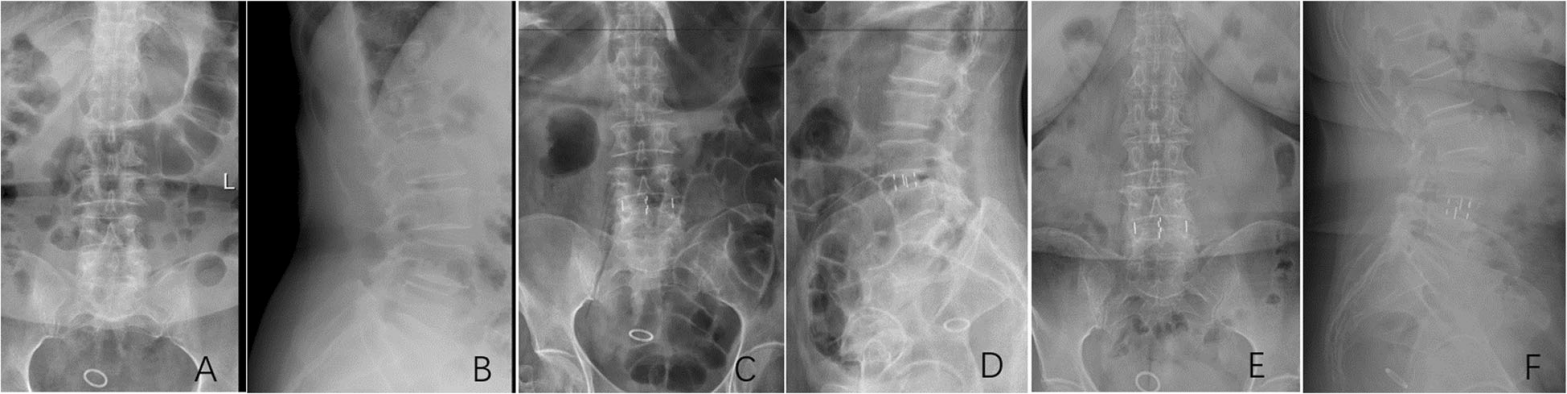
A 63-year-old woman suffered from low back and bilateral lower limb pain for 6 years, aggravating for 4 months. X-ray of the lumbar spine showed a typical case of single-level OLIF for L4 degenerative spondylolisthesis. a Preoperative anteroposterior radiograph. b Preoperative lateral radiograph. c Postoperative anteroposterior radiograph. d Postoperative lateral radiograph. e The last follow-up anteroposterior radiograph. f The last follow-up lateral radiograph
